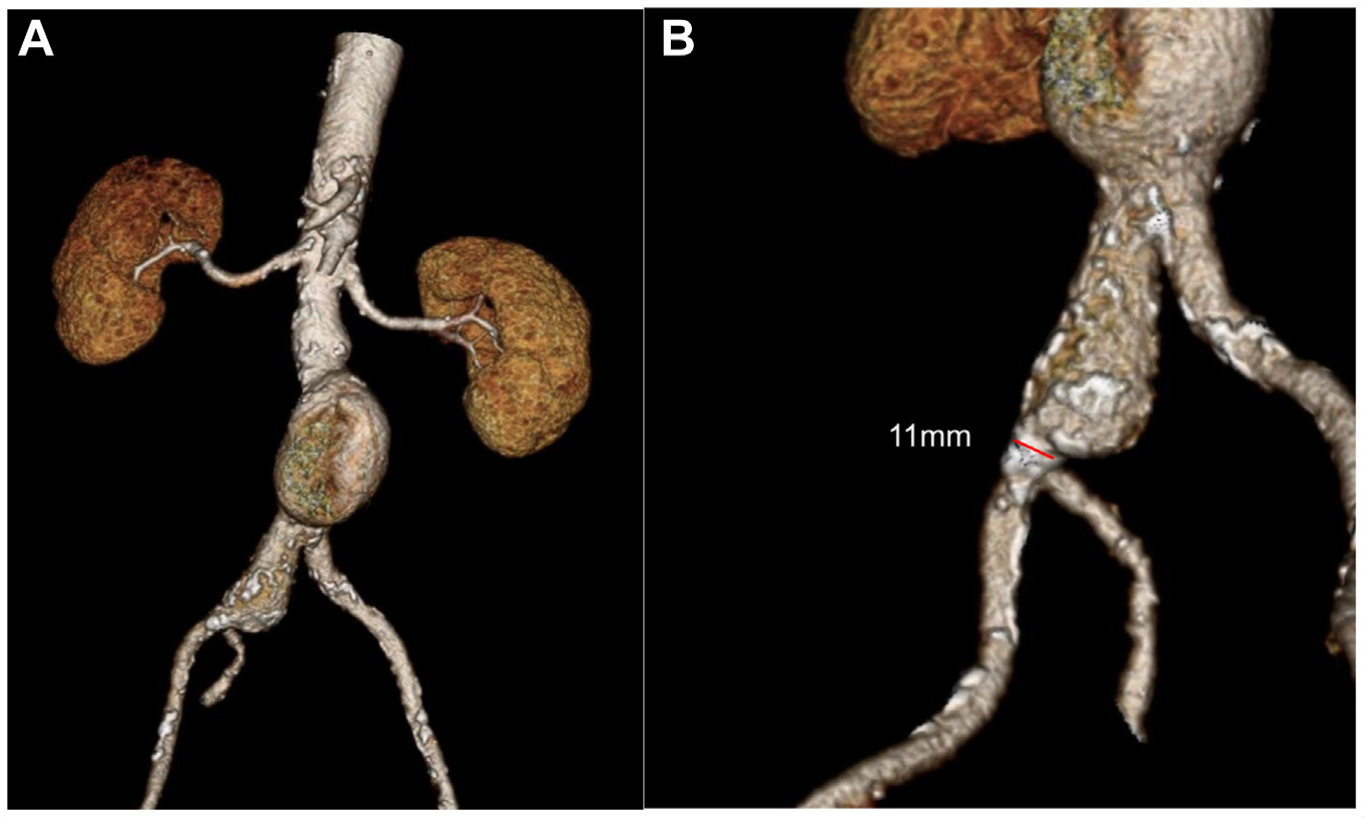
Preoperative computed tomography angiography (CTA) three-dimensional reconstruction shows the aortic saccular aneurysm (A). The right common iliac artery presented some anatomical features that could expose to patient to higher risk of type IB endoleak: its maximum diameter was 24 mm with a conical shape. It also presented a narrowing to 11 mm just before the hypogastric artery origin (B).
